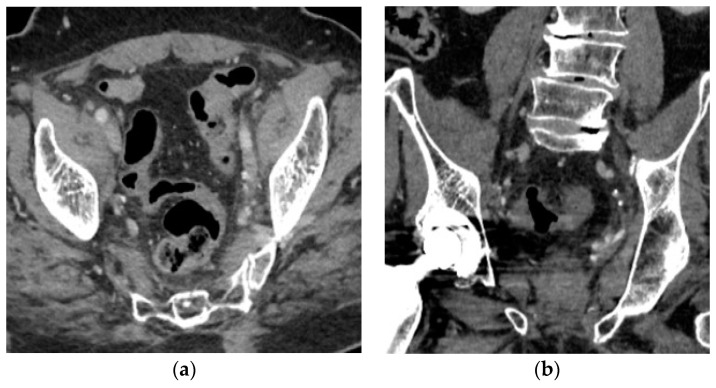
Six months follow-up contrast-enhanced CT scan: (a) axial view and (b) coronal view of the pelvis that showed no signs of recurrence or progression.
